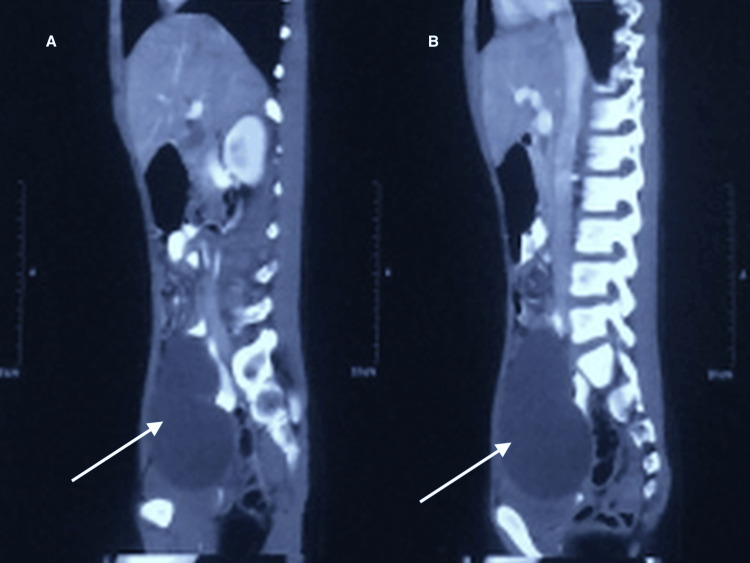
CECT abdomen and pelvis CECT: contrast-enhanced computed tomography CECT scan of the abdomen showing a large, multiloculated, well-marginated cystic lesion in the abdominopelvic region, initially suspected to be of paraovarian origin. Panels A-B showing saggital sections depicting large cystic lesion involving both abdomen and pelvis
